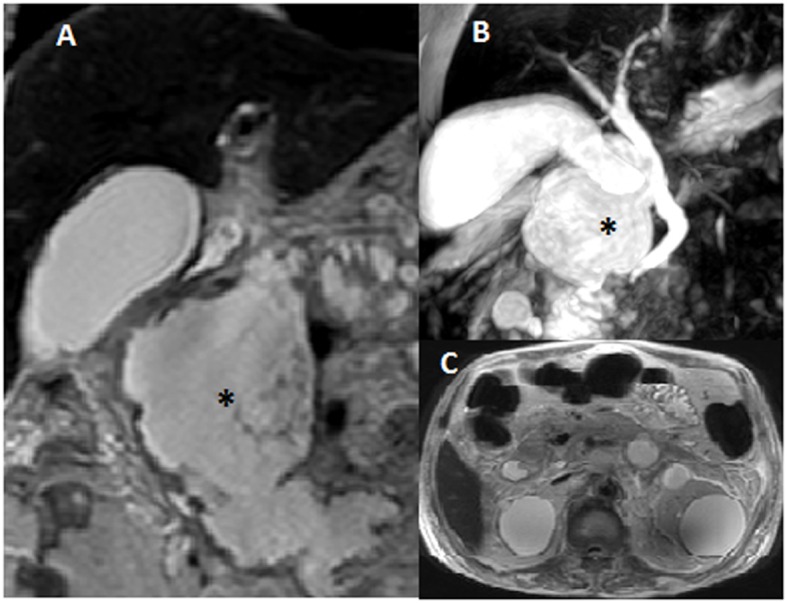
Magnetic resonance cholangiopancreatography with MRI abdomen with pelvis showed large 8 × 5 × 5 cm cystic irregular collection (asterisk) with solid component and septations involving head and body of the pancreas, almost replacing them (A, B). Bilateral multiple cysts in kidneys were also noted (C).
